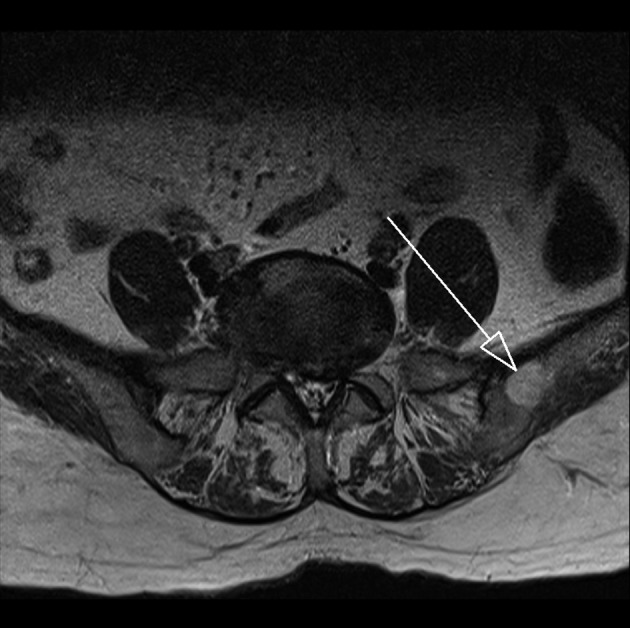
Magnetic resonance imaging showing the left ilium with an abnormal signal.
